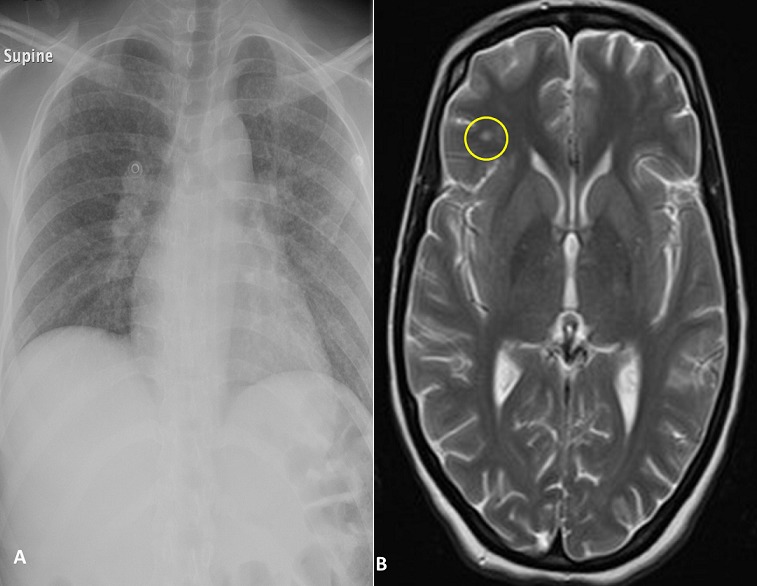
(A) chest X-ray showing bilateral hilar opacities, with suspicious miliary infiltrates; (B) brain MRI showing hyper-intense foci in left frontal lobe brain matter
